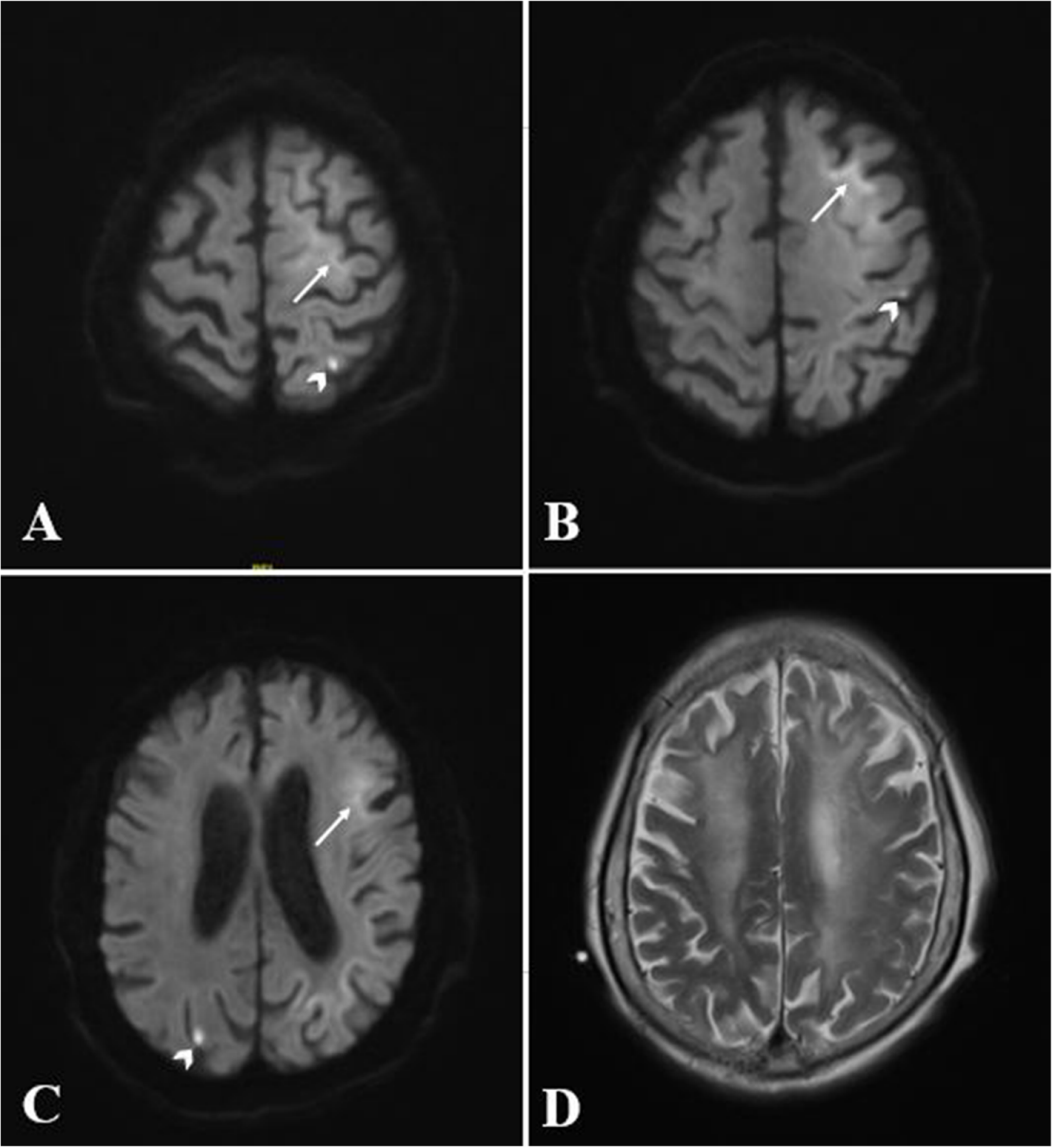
Brain MRI findings in an 86-year-old patient with NIID. Brain MRI reveals asymmetrical, curved, high signals along the corticomedullary junction on DWI (arrow, A, B and C), punctate high intensity signals in the cortex on DWI (arrowhead, A, B and C), and diffuse high signals of white matter on T2-weighted images (D)
